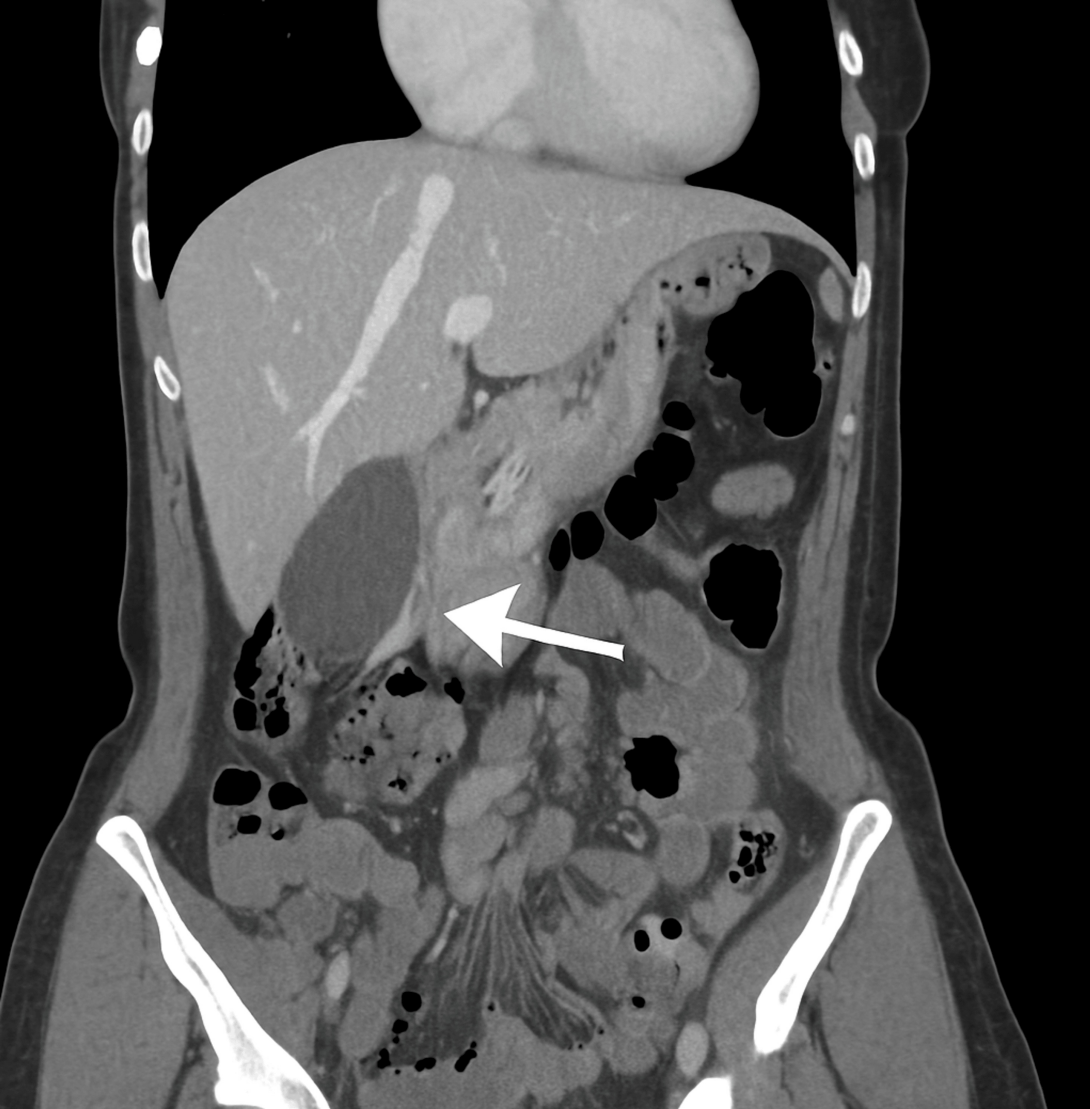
Coronal CT scan showing mild pancreatic edema This coronal view highlights the edematous pancreas (white arrow) without evidence of necrosis or pseudocyst formation.
